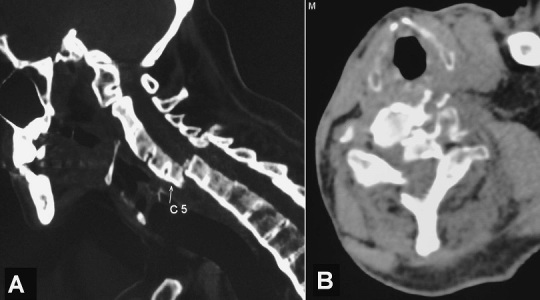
Cervical computed tomography scan on sagittal (A) and axial (B) views revealing ossification of the anterior longitudinal ligament, calcification of the intervertebral discs and complete vertebral fusion (bamboo spine) with transdiscal fracture at C5-C6 level causing a luxation of the cervical spine with significant compromise in canal space
